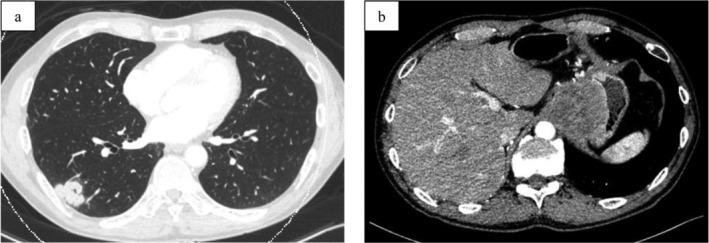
Computed tomography (CT) scan. (a) Chest scans indicated the presence of a right lower lobe nodule which measured 3.2 × 2.3 cm. (b) Abdominal scans showed a gastric fundal mass located in the gastric cardia which measured 5.2 × 5.0 cm.
